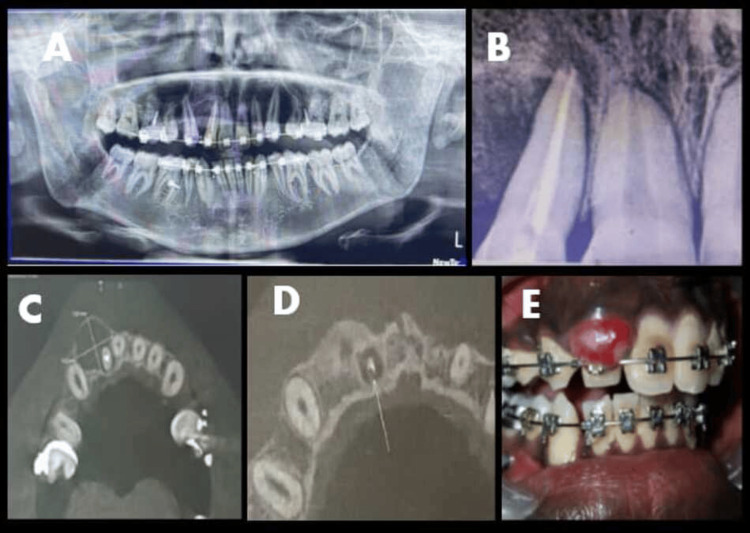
(A) Preoperative orthopantomogram. (B) Periapical radiograph showing a root canal-treated tooth in 12. (C, D) Cone-beam CT images showing expansion of the buccal cortical plate. (E) Intraoral photograph showing swelling in the labial region of 12.
